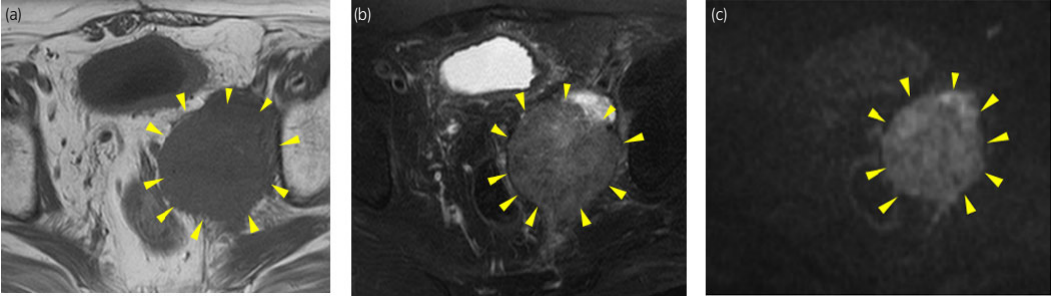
MRI showed that the mass with arrow pointing was isointense on (a) T1‐ and (b) T2‐weighted imaging and hyperintense on (c) diffusion‐weighted imaging.
